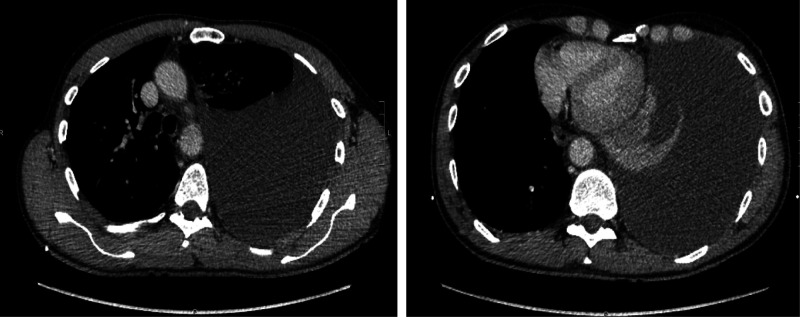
CT scan showing a uniform left massive pleural effusion.
